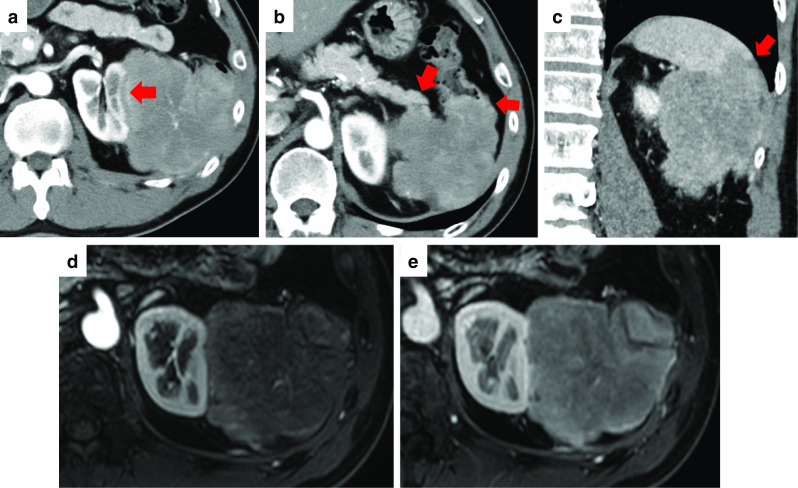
a–c Abdominal contrast-enhanced CT scan showed a huge mass in the spleen. The red arrows point out the involvement of surrounding organs: a left kidney, b pancreatic tail and descending colon, c left diaphragm. d Early phase of abdominal contrast-enhanced MRI. e Late phase of abdominal contrast-enhanced MRI
